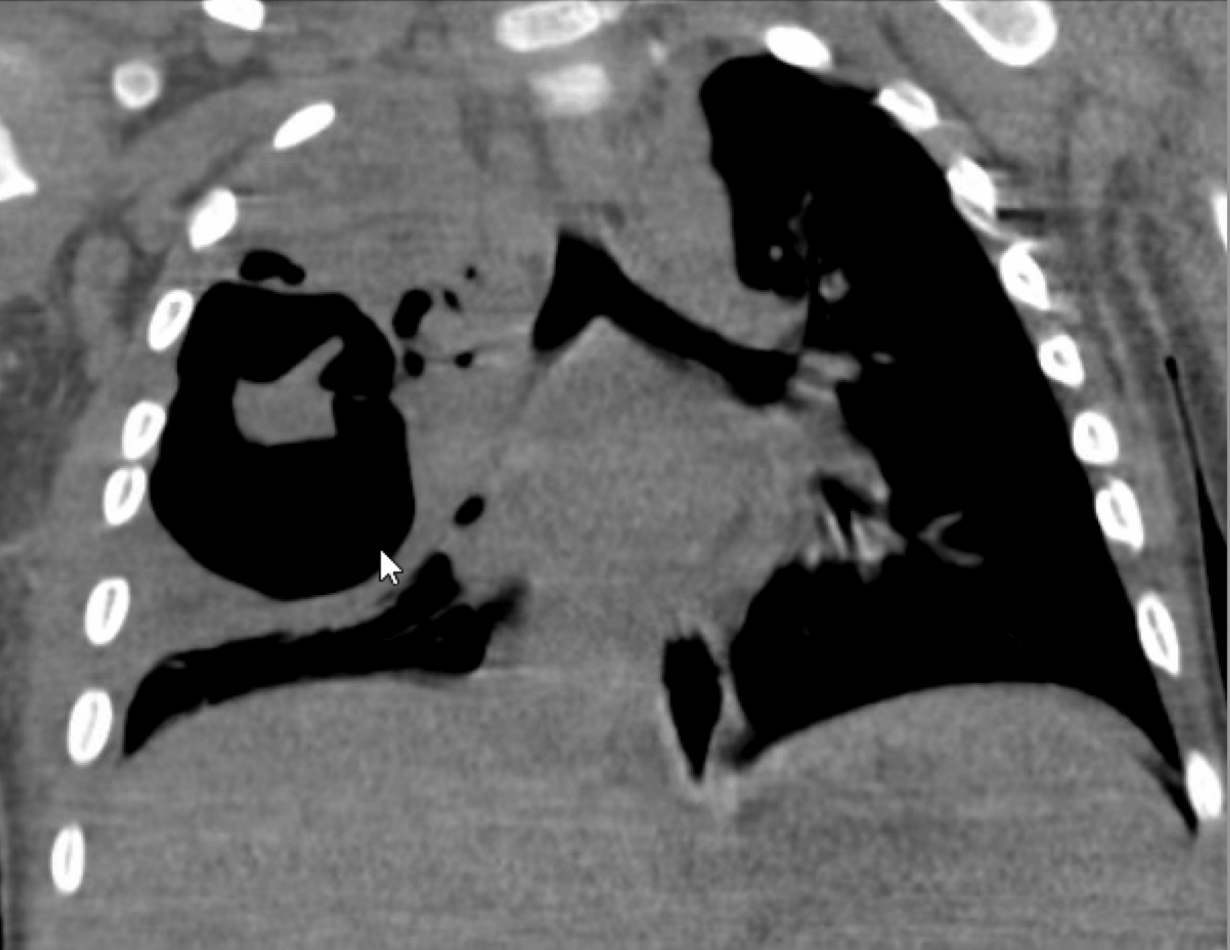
CT chest (coronal view), hospital day 1; large thick-walled cavity in the right upper lobe with surrounding smaller cavitary lesions.
